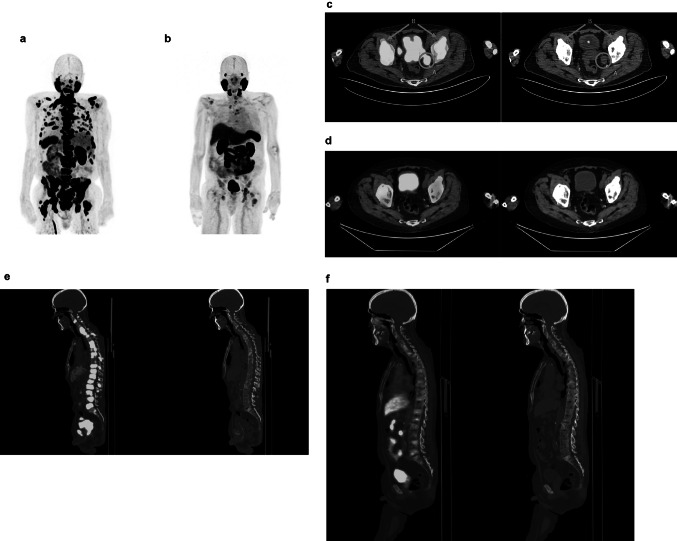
a) 3D MIP of a Gallium-68 PSMA PET scan at time of diagnosis showing numerous foci of PSMA avid uptake within the prostate mass, supraclavicular, retroperitoneal, and pelvic lymph nodes, and the axial and proximal appendicular skeleton. b) 3D MIP of a Gallium-68 PSMA PET scan 16 months following initial scan, after treatment with PSMA-Lu177, showing marked improvement of previously visualized areas of PSMA avid uptake within the prostate mass, supraclavicular, retroperitoneal, and pelvic lymph nodes, and the axial and proximal appendicular skeleton.c) Axial PSMA PET/CT (left) and CT soft tissue window (right) at time of diagnosis showing avid PSMA uptake within a 2 cm short axis left pelvic sidewall lymph node (A, circled) and the bilateral pelvic bones (B, arrows).d) Axial PSMA PET/CT (left) and CT soft tissue window (right) 16 months after initial scan showing resolution of the previously visualized 2 cm short axis left pelvic sidewall lymph node and improvement of PSMA uptake within the bilateral pelvic bones.e) Sagittal PSMA PET/CT (left) and CT bone window (right) at time of diagnosis showing diffuse avid PSMA uptake within the spinal vertebral bodies and posterior elements with associated mixed lytic and sclerotic lesions, reflecting diffuse osseous metastatic disease.f) Sagittal PSMA PET/CT (left) and CT bone window (right) 16 months following initial scan showing interval decrease in intensity of PSMA uptake within the spinal vertebral bodies and posterior elements with associated persistent mixed lytic and sclerotic lesions.
